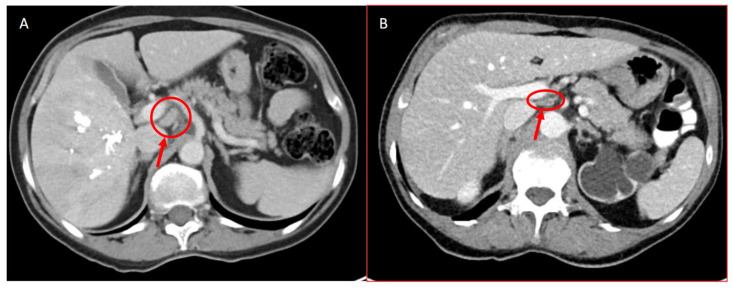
CT scans of patients with LN metastasis. (A) shows a CT scan of a LN+ patient with an already on-imaging suspicious LN (red arrow). (B) shows a CT scan of a LN+ patient where the LNs on imaging were not suspicious (red arrow).
